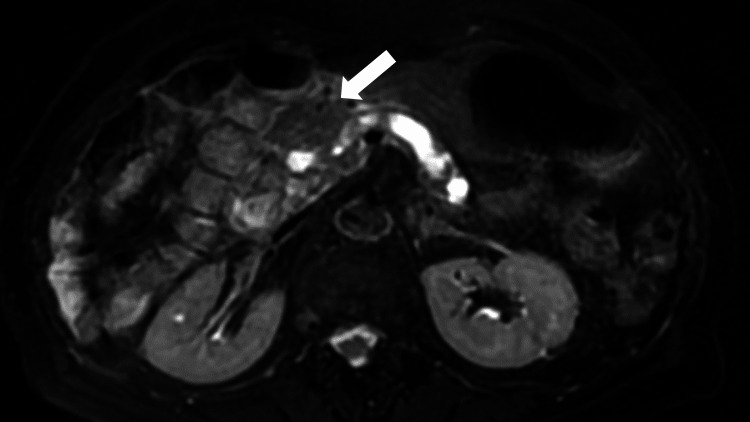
Magnetic resonance image showing a decreasing trend of findings of acute pancreatitis in the pancreatic head with mild swelling in the pancreatic head (white arrow)
